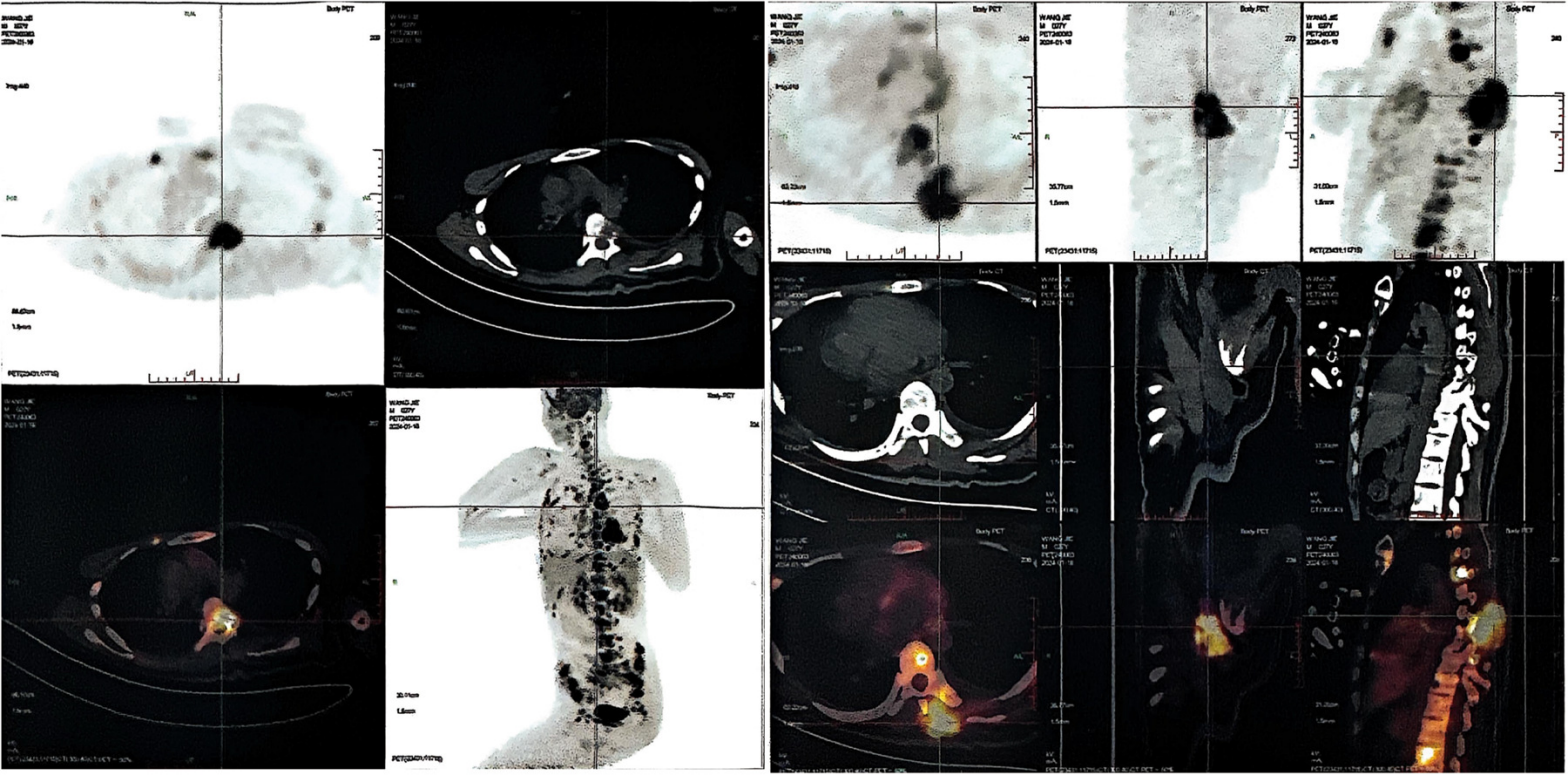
Positron emission tomography-computed tomography (PET-CT) imaging in patients with advanced stage tumors. Whole body PET-CT imaging showed multiple bone metabolic abnormalities, most notably in the spine, bilateral ilium and bilateral ribs.
